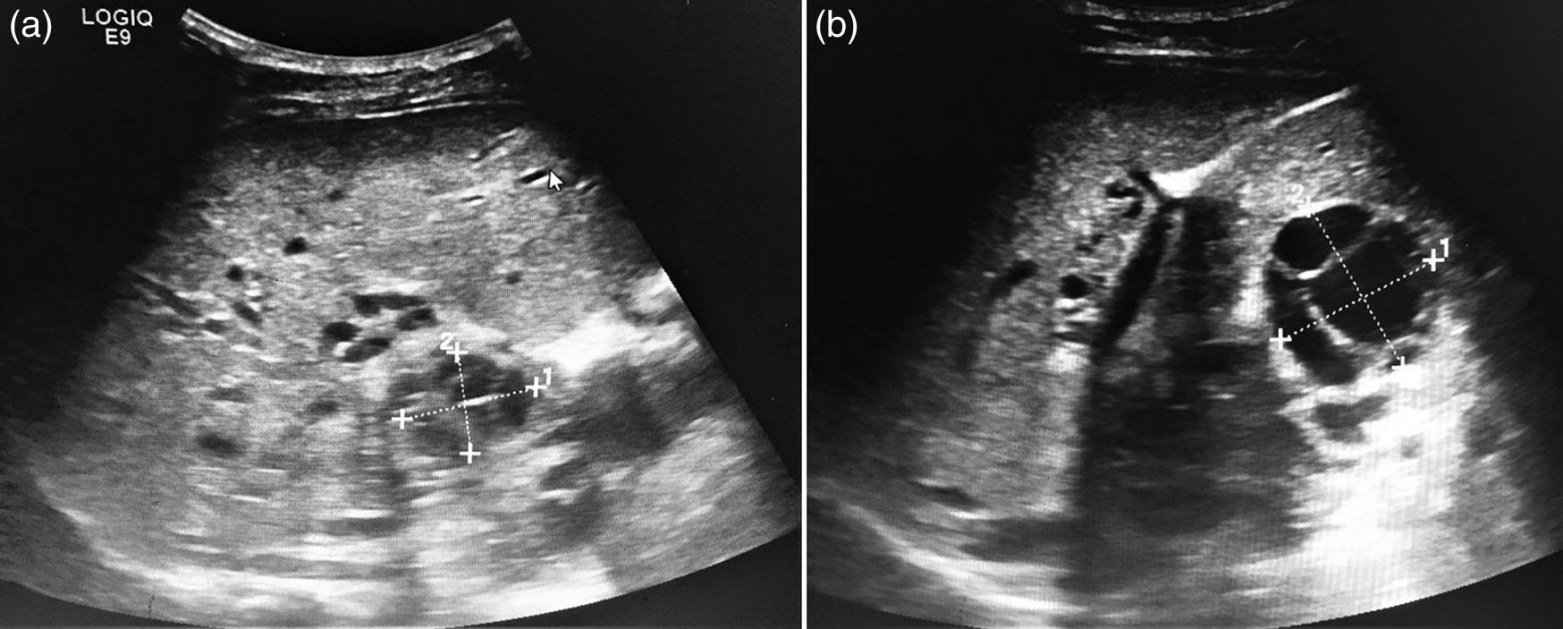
a and b: An abdominal ultrasound showing the presence of multiple multiloculated cystic formations evoking hydatic cysts type CE2 of the WHO classification of segments I, IV, and V.
